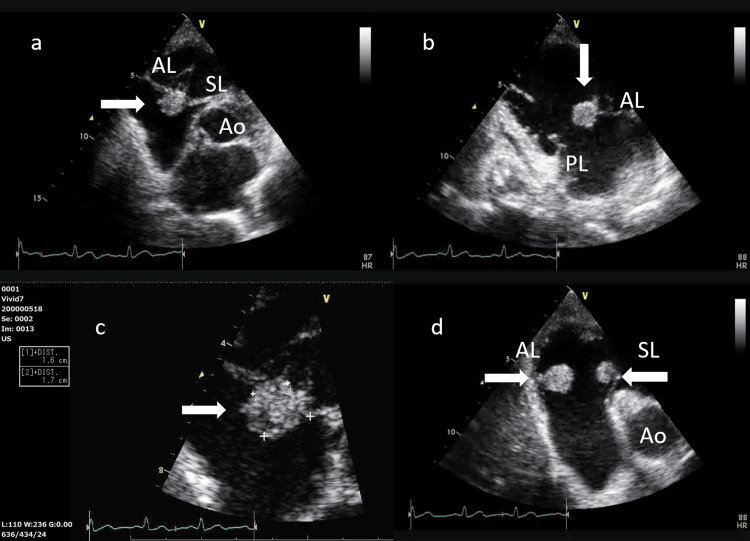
TTE (a to d) a: Arrow demonstrates the vegetation between the anterior tricuspid leaflet and the septal tricuspid leaflet during ventricular systole. b: Arrow shows that the vegetation involves only the anterior tricuspid leaflet. No vegetation is seen on the posterior tricuspid leaflet during ventricular diastole. c: The size of the vegetation was 16 mm × 17 mm during ventricular systole. d: Arrows showed separate vegetation on both the anterior tricuspid leaflet and the septal tricuspid leaflet during ventricular diastole. TTE: transthoracic echocardiography, Ao: aorta, AL: anterior tricuspid leaflet, PL: posterior tricuspid leaflet, SL: septal tricuspid leaflet
